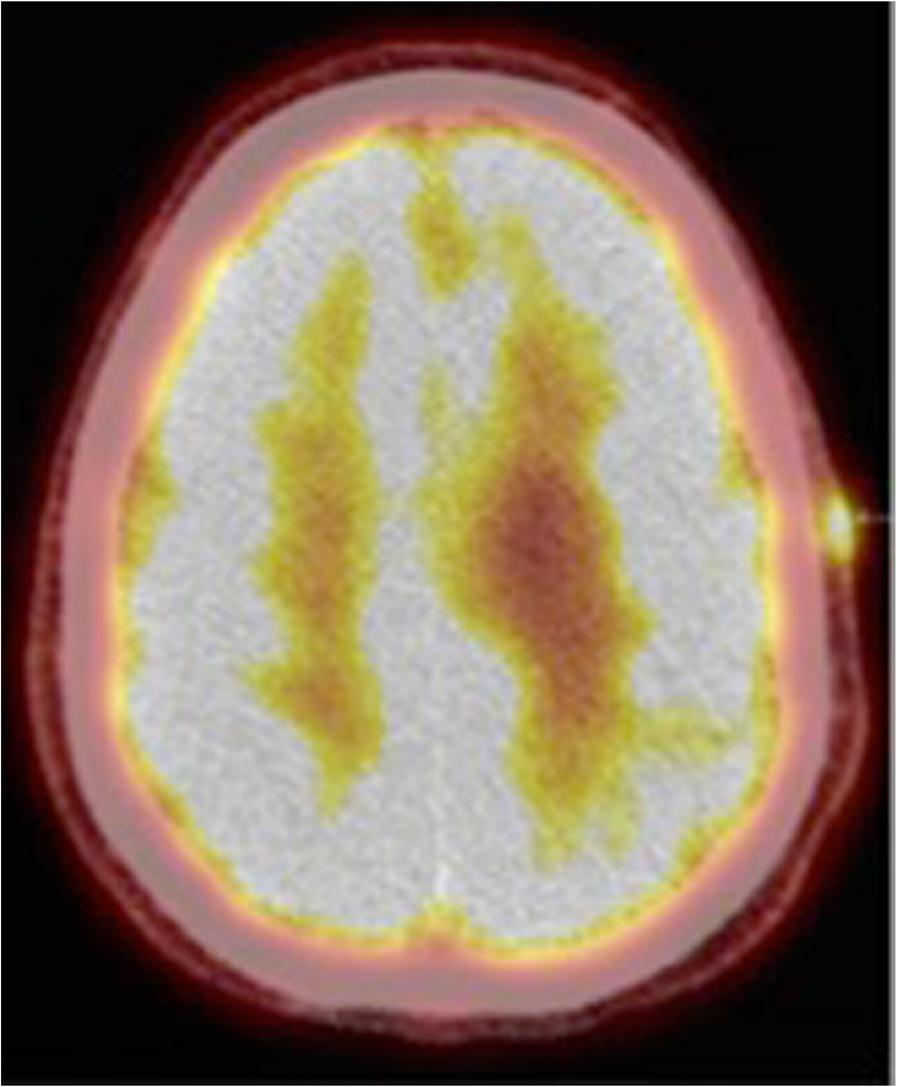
PET-CT demonstrating local recurrence in the left scalp
